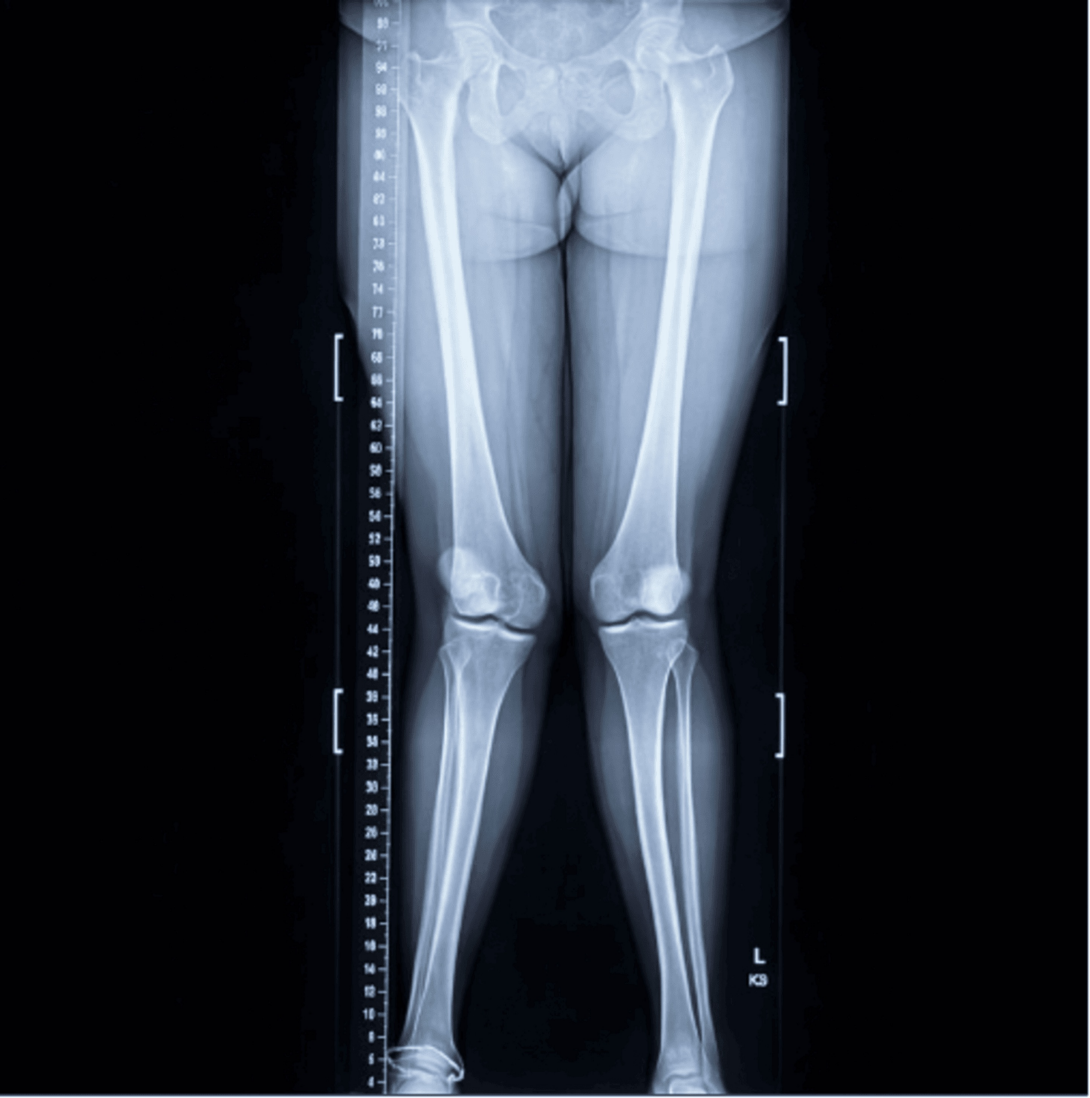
Full-length standing anteroposterior lower-extremity radiograph demonstrating the patient's genu valgum (knock-knee) deformity
